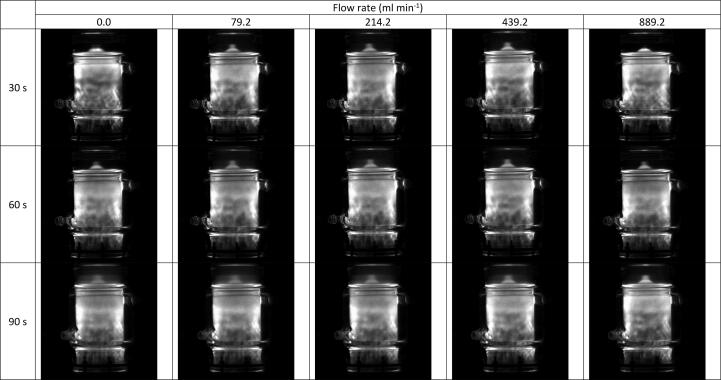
Sonochemiluminescence images for the sonolysis of 420 ml of Milli-Q water using 410 kHz ultrasound at 200 W L^−1^ applied power, at liquid flow rates of 0.0, 79.2, 214.2, 439.2, and 889.2 ml min^−1^, left to right respectively, and times of 30, 60, and 90 s, top to bottom respectively.
